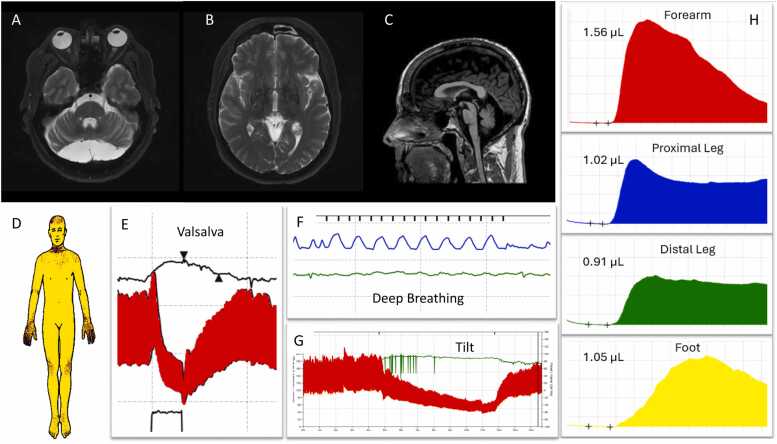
Axial T2 MRI demonstrates a retrocerebellar cyst (A) and putaminal rim sign (B). Sagittal T1 shows pontine and cerebellar peduncle atrophy (C). TST shows global anhidrosis (94.5 %) in a central pattern (D). There is severe cardiovascular adrenergic impairment with Valsalva (E) and deep breathing (F). Tilt shows neurogenic orthostatic hypotension (G). Postganglionic sweating is normal (H).
